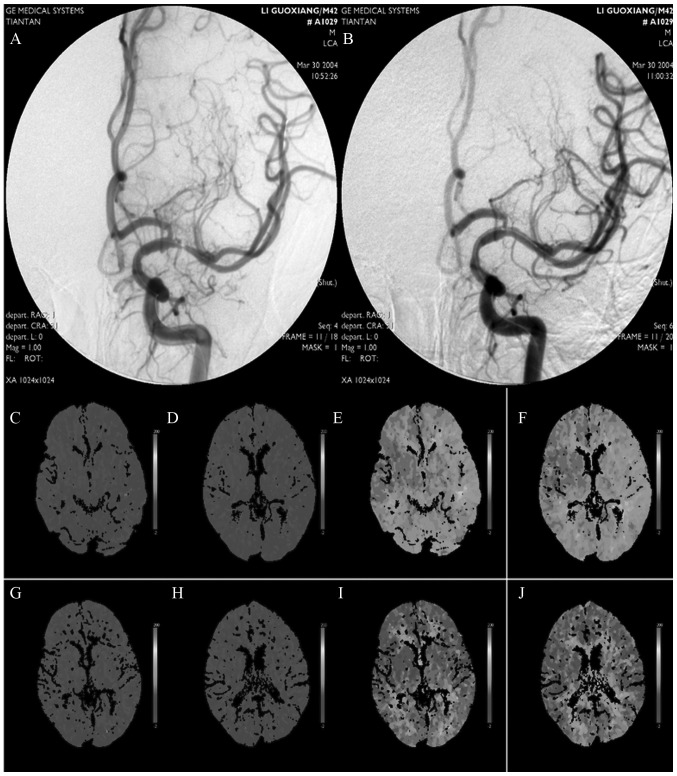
DSA and CT images of patients with middle cerebral artery stenosis. (A) Preoperative stenosis rate of 99% at the M1 segment of the LMCA by DSA; (B) no notable postoperative residual LMCA stenosis in the same patient by DSA; (C–F) preoperative prolonged MTT and TTP at the left frontal lobe, left temporal and bilateral occipital lobes, respectively, by cranial perfusion CT; and (G–J) postoperative, essentially normal MTT and TTP at the left frontal and temporal lobes but high MTT and TTP at the bilateral occipital lobes. LMCA, left middle cerebral artery; DSA, digital subtraction angiography; MTT, mean transit time; TTP, time-to-peak.
